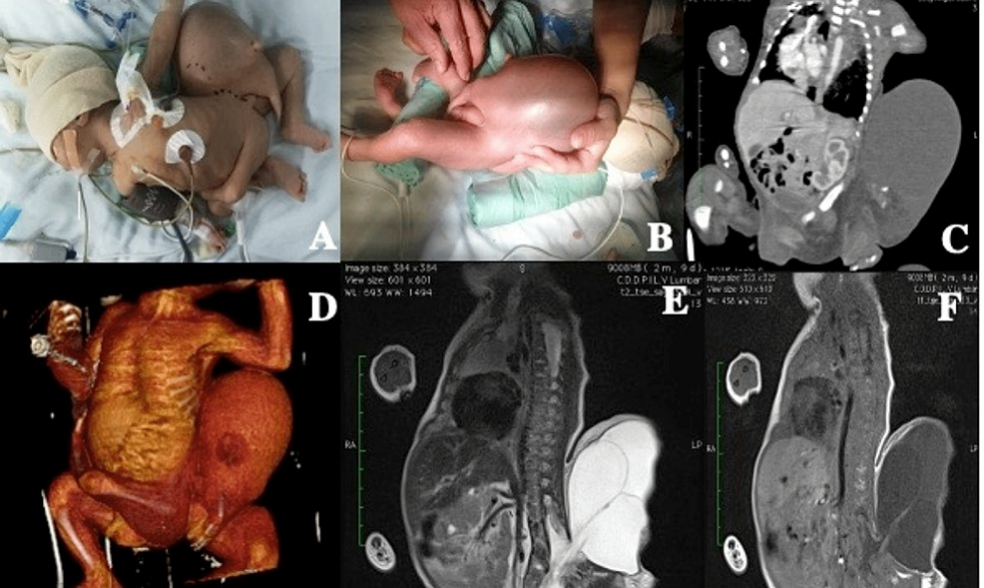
A and B: A one-week-old newborn in good general condition with paraplegia (T12-L1) with a parasitic twin attached from the dorso-lumbosacral region, amorphous, that contains rudimentary traces of the hand, right finger and left finger outline, and two extremities. C and D: Simple tomographic images and 3D reconstruction where the amorphous parasite twin with a liquid cavity and part of the extremities adhered to the newborn with the organs, viscera, muscles, and bones of the latter with adequate biology and formation. D and E: Magnetic resonance images in T1 and T2 where it is observed that both share the same column, spinal defect of the posterior elements from L1 to L2 onward, compatible with spina bifida with nerve root involvement and with a lesion compatible with myelomeningocele with multiple septal agenesis of almost the entire sacrum with a discreet vestige.
